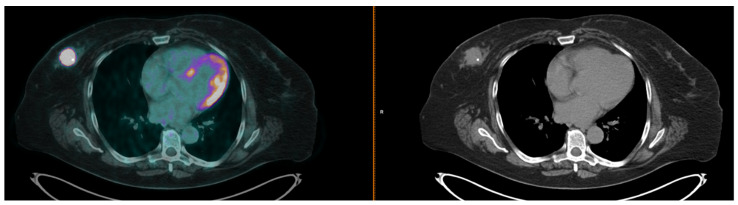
Transaxial fused (on the left) and CT images (on the right) of a baseline [^18^F]FDG PET/CT performed in a patient with TNBC, before starting NAC. A focal area of uptake can be seen in the right breast tumor. On CT images, a clip positioned within the lesion during the biopsy can be observed.
